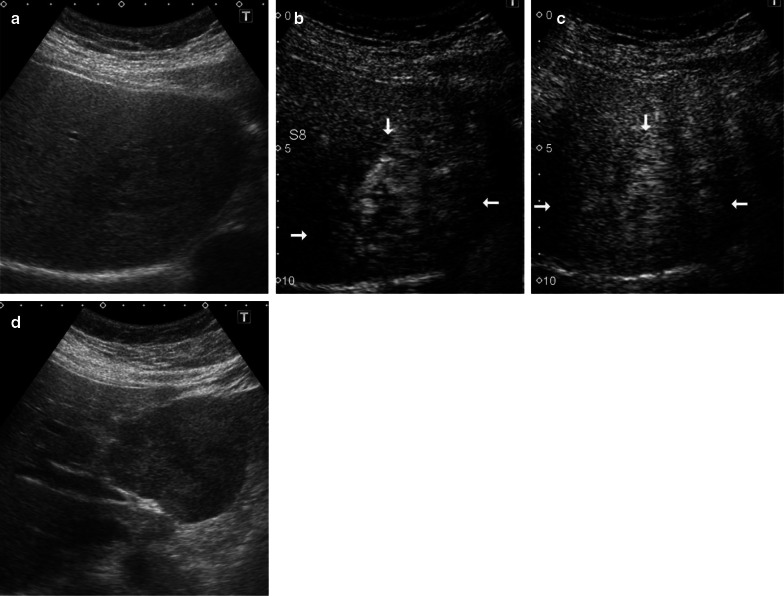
Sonographic findings. a–c Brightness mode and contrast-enhanced sonography reveal liner structures (white arrow) in liver segment IVa. d A 78-mm, hyperechoic mass in liver segment IVb has a hypoechoic mixed central region
